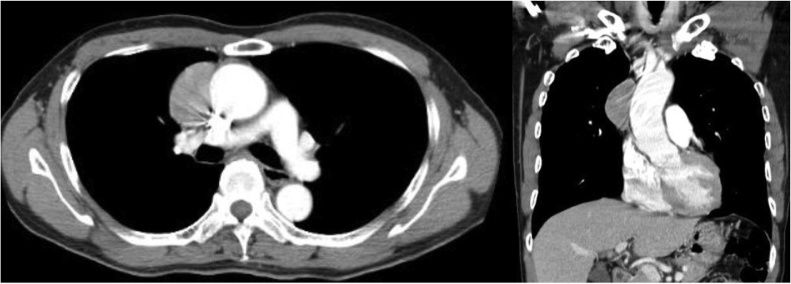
Contrast computed tomography scan of the chest: 47-mm solid mass with a clear rim in the anterior mediastinum.
